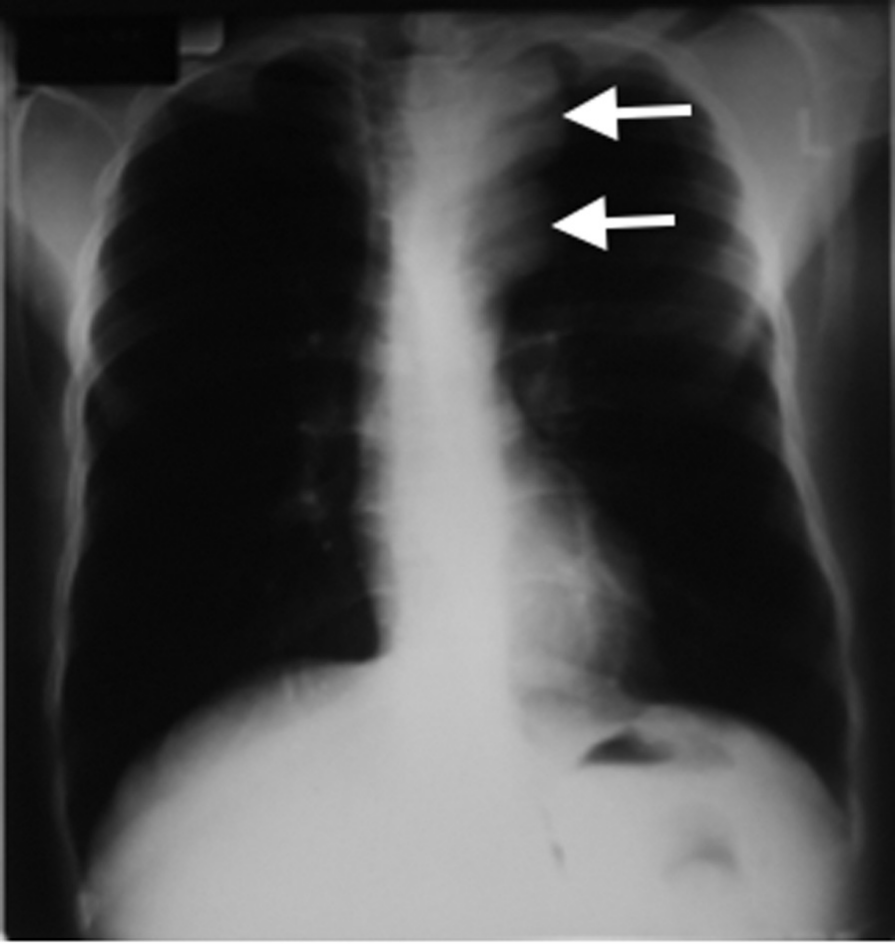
Chest radiograph showing superior mediastinal mass (arrows) and tracheal deviation.
